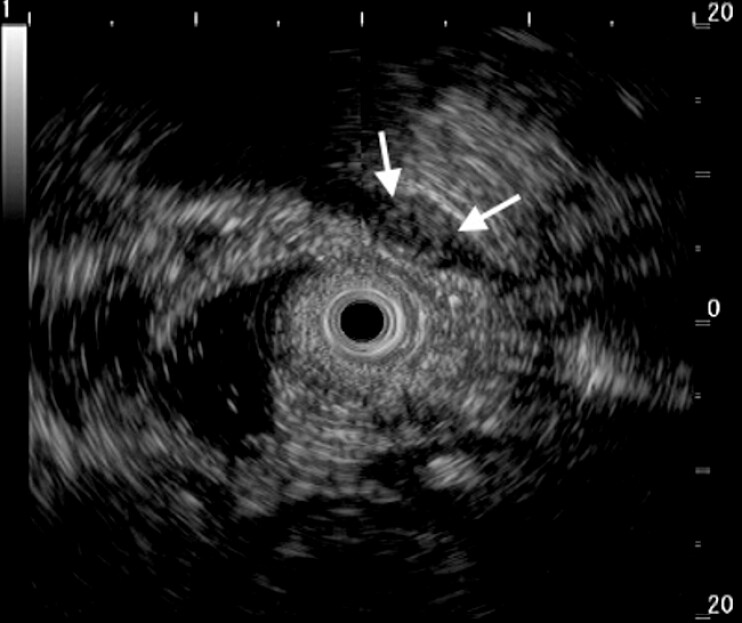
Endoscopic ultrasonography image using a miniprobe showing a 6 × 4-mm hypoechoic mass originating from the muscularis propria.
